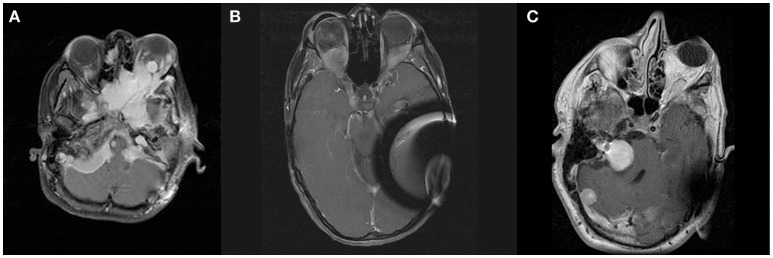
Panel (A) shows contrast enhanced T1-weighted axial images at the level of the orbits demonstrating meningiomas of skull base, left orbit, and right orbital apex of patient A. Panels (B,C) show contrast enhanced T1-weighted axial images at the level of the orbits demonstrating orbital apex meningioma of patients B and C, respectively.
